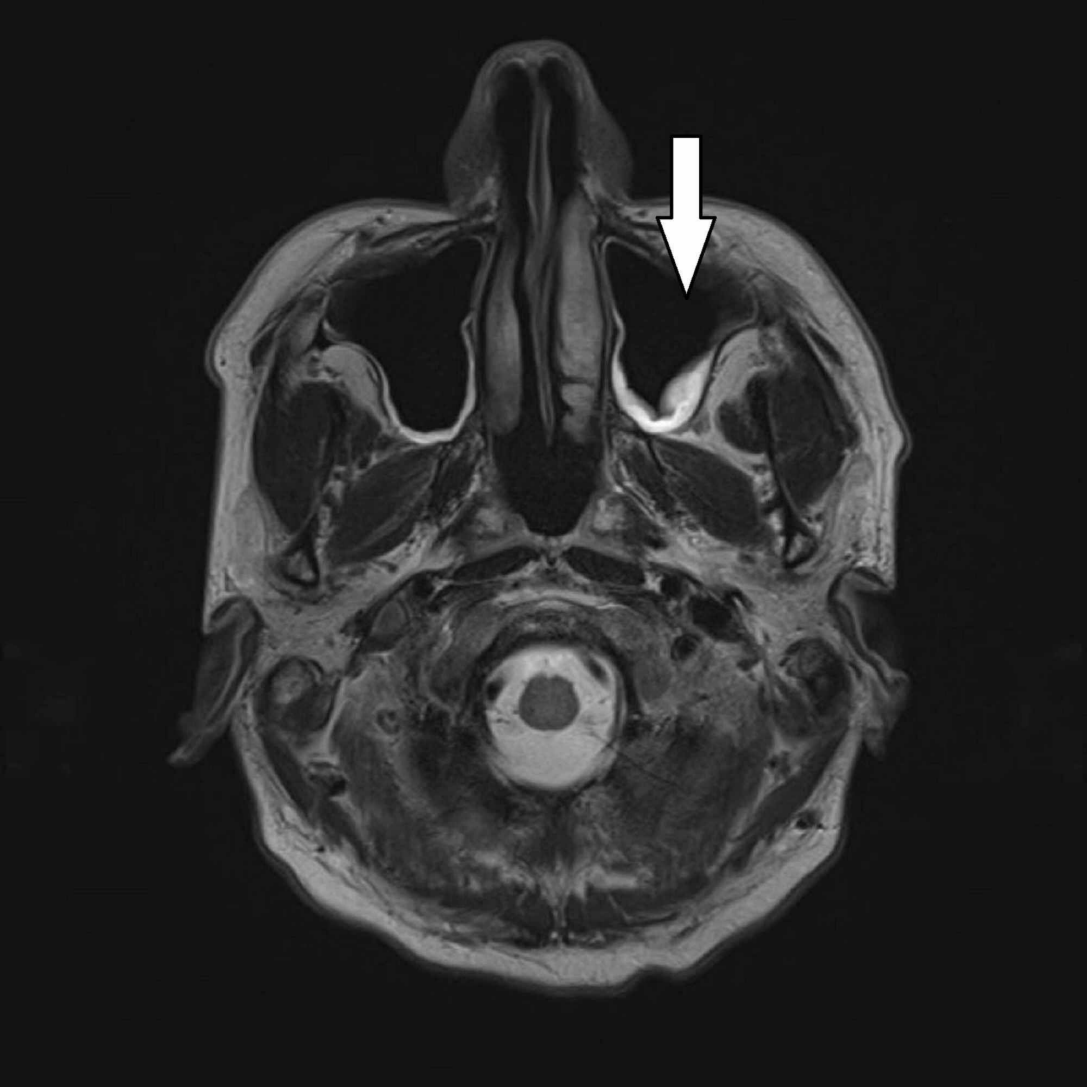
MRI demonstrating sinusitis
